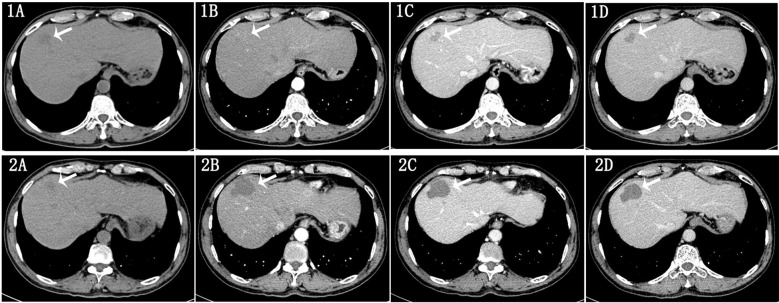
Male, 60 years old, diagnosed with hepatocellular carcinoma (HCC) in liver segment S8 via clinical/pathological biopsy. (A–D) show contrast-enhanced CT scans performed 1 week before ablation therapy. (A) Non-contrast scan shows a hypodense lesion. (B) Arterial phase reveals mild heterogeneous enhancement of the lesion. (C) Venous phase demonstrates washout of contrast. (D) Delay phase shows further decrease in enhancement. Images 2A–D show follow-up contrast-enhanced CT scans 29 weeks post-ablation therapy: Image 2A: Non-contrast scan shows a hypodense lesion. Image 2B: Arterial phase shows no significant enhancement. Images 2C, D: Venous and delayed phases confirm persistent absence of enhancement. Conclusion: Complete resolution of the lesion after ablation therapy.
